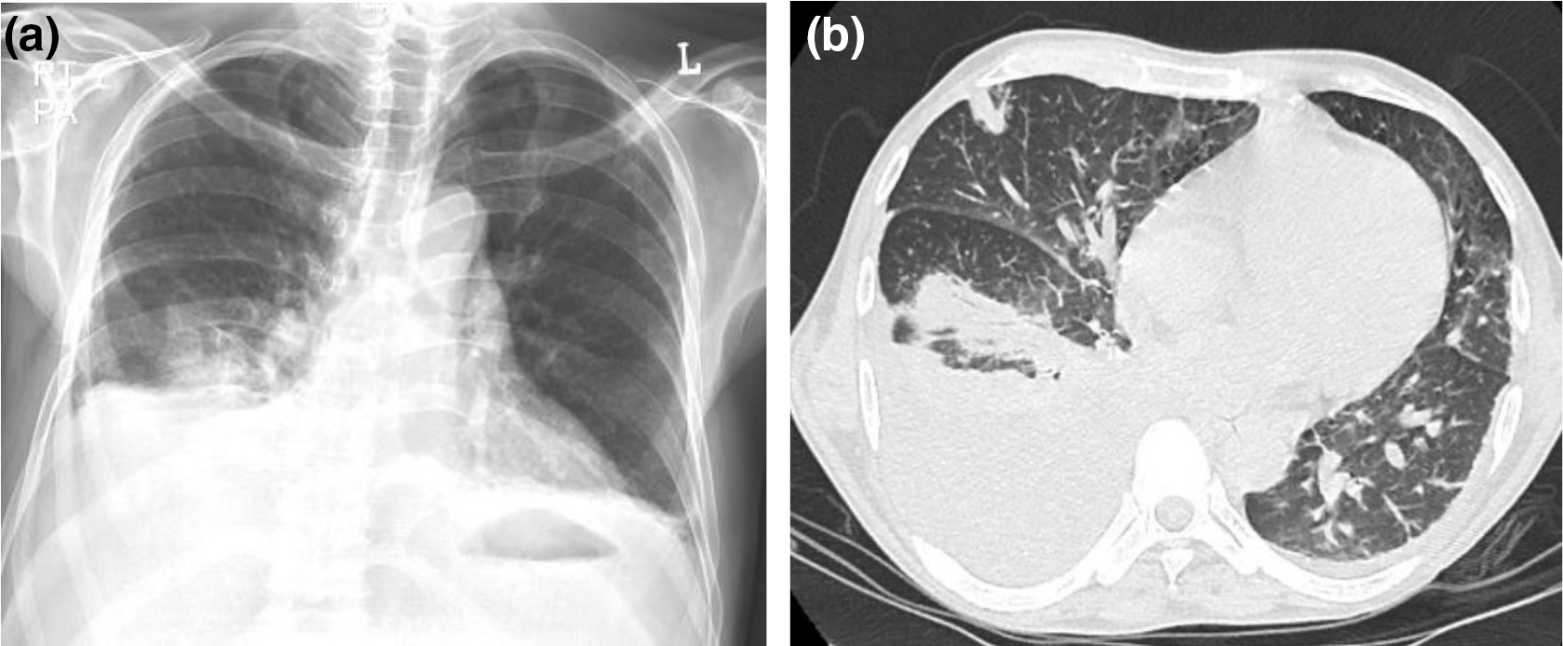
(a) Chest X-ray (PA view) showing pleural effusion and (b) HRCT of the chest (axial view) for case 1 depicting multiple nodules.
